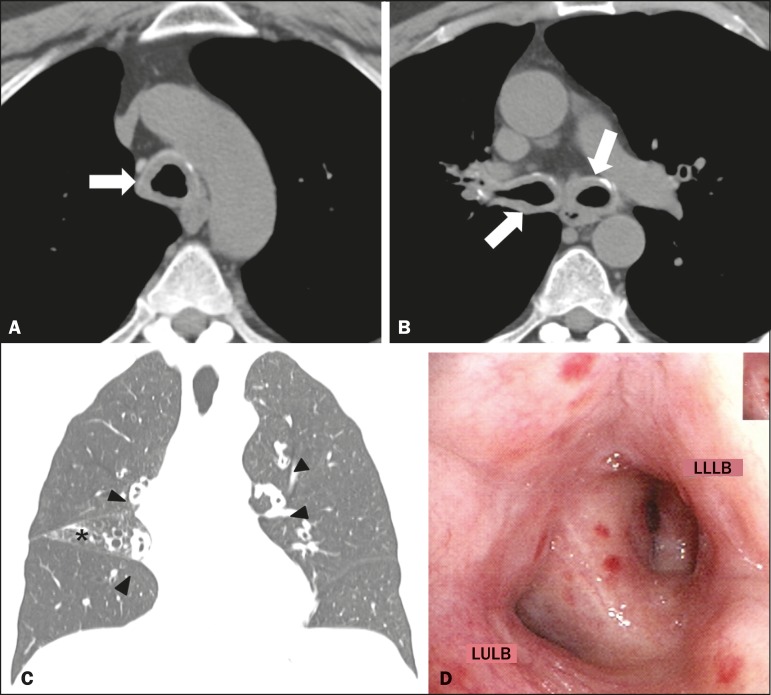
A,B: Axial CT scan of the chest (mediastinal window), without
